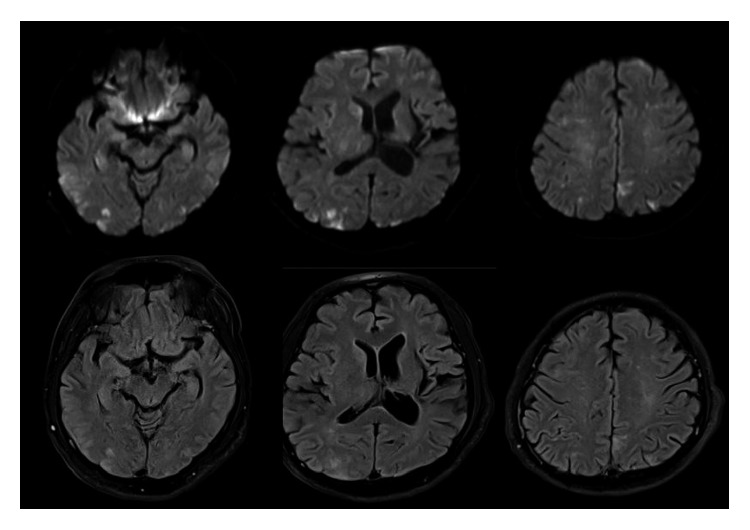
MRI brain DWI (upper) and FLAIR (lower).
